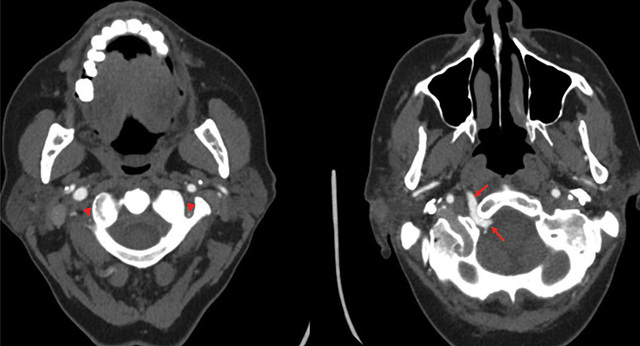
Axial CT-angiogram images demonstrating hypoplastic vertebral arteries (arrowheads) and a right persistent hypoglossal artery (arrows) coursing through a widened hypoglossal canal.
